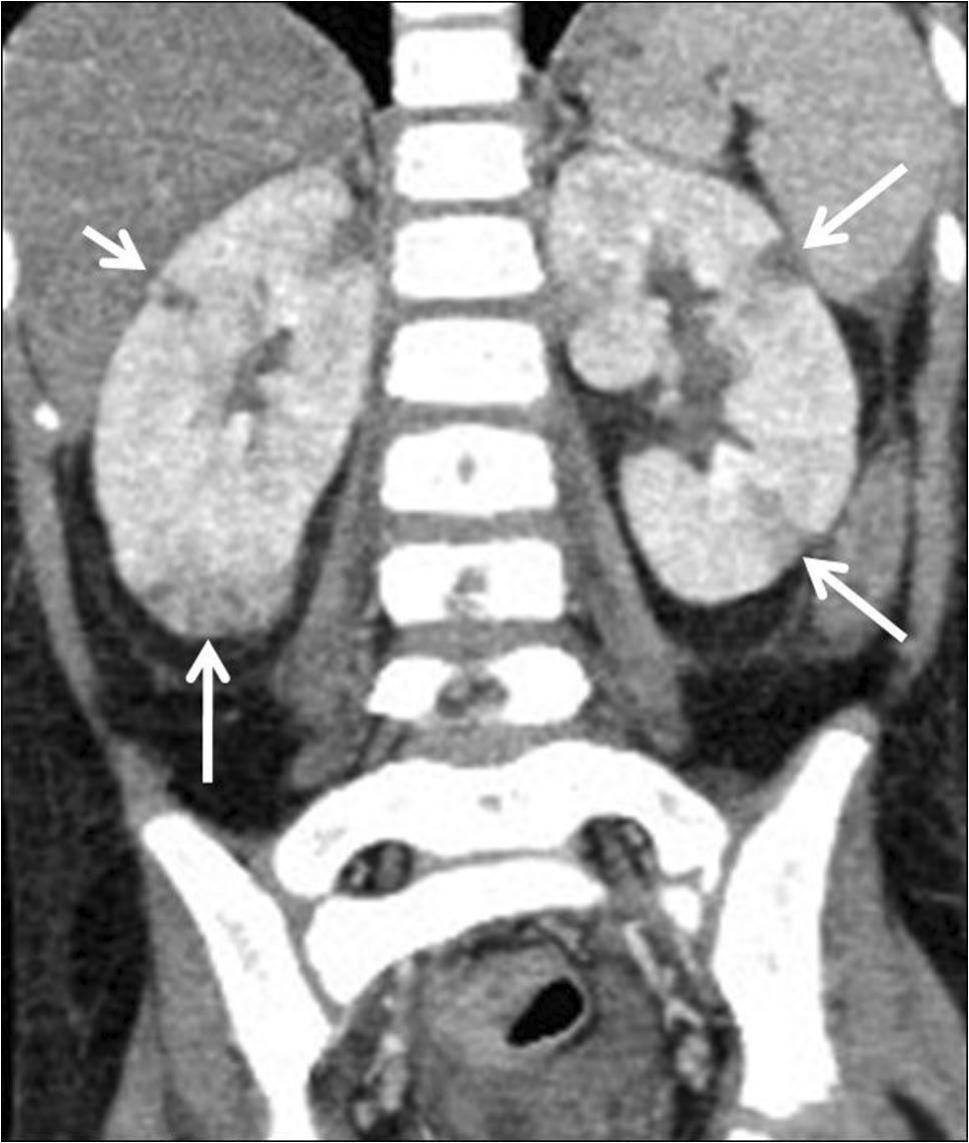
Tuberculous pyelonephritis in a 4-year-old boy. A coronal contrast-enhanced computed tomography image of the abdomen shows a striated nephrogram in the bilateral kidneys with wedge-shaped hypodense areas (*arrows*)
